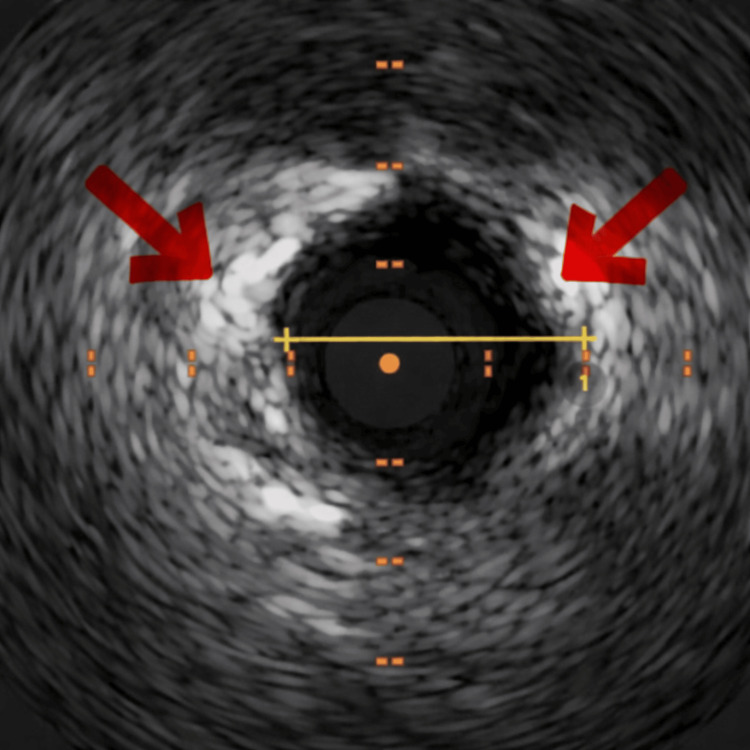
IVUS Demonstrating Absence of Plaque Rupture IVUS demonstrating mild coronary atherosclerosis without evidence of plaque rupture, erosion, or dissection. Red arrows highlight areas of atherosclerotic plaque. IVUS: intravascular ultrasound
